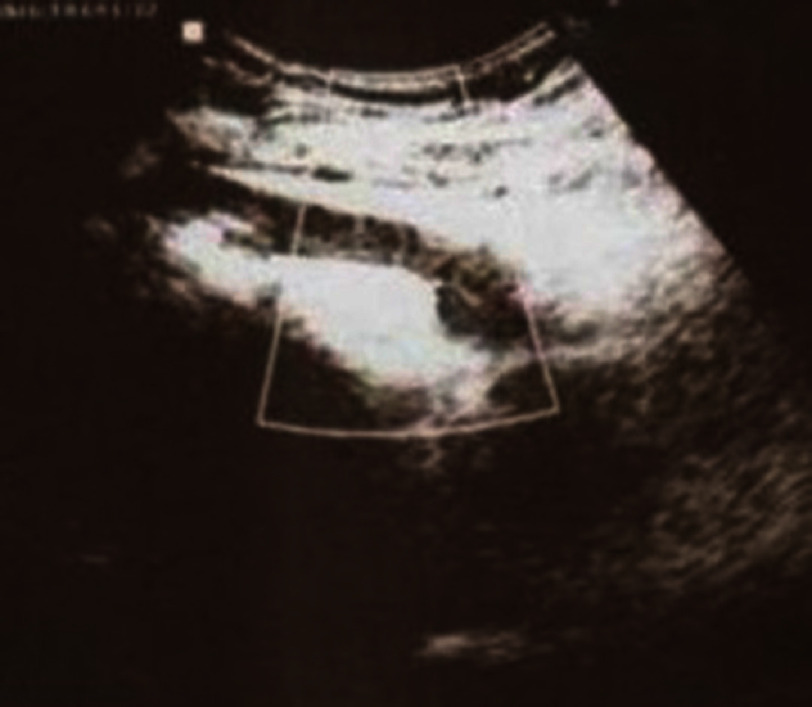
Doppler ultrasound showing thrombotic and non-compressible saphenous vein.
